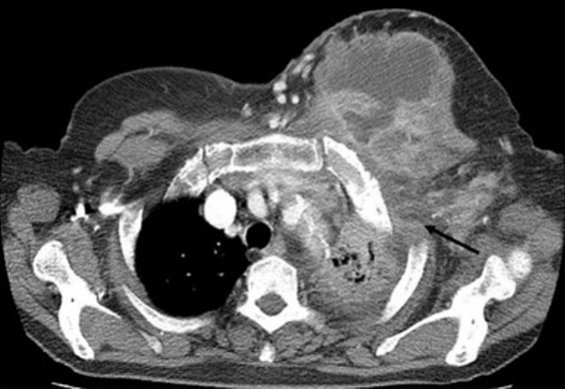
Actinomycosis involving the chest wall in a 75-year-old woman, manifesting as a palpable mass on the left chest wall. Axial CT image shows a heterogeneous mass with central low attenuation and peripheral enhancement on the left chest wall, contiguous with consolidation (*arrow*) in lung parenchyma, and pleural effusion
